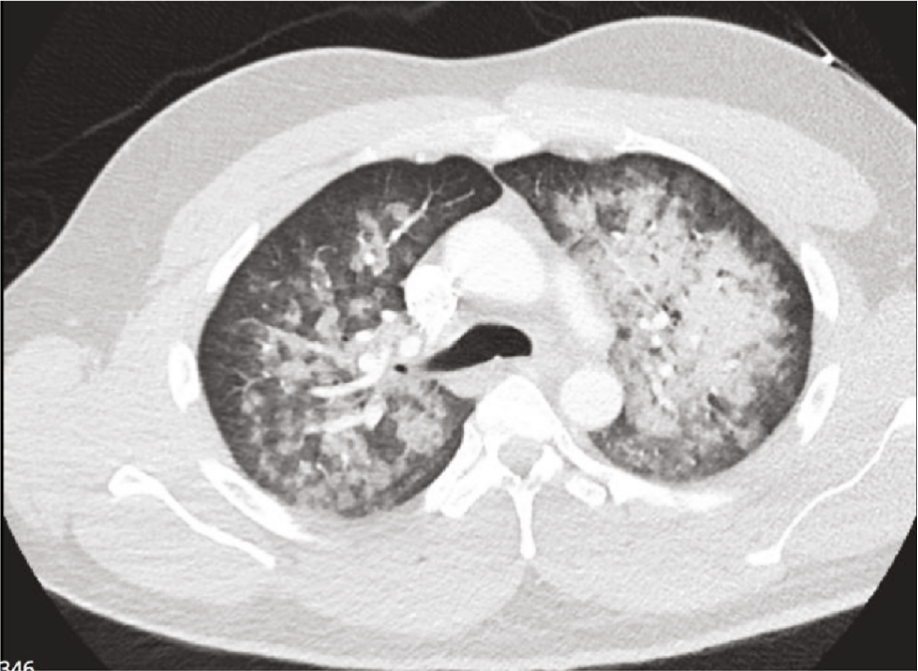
Contrast-enhanced computed tomography of the chest on day #2 shows diffuse bilateral airspace disease characterized by groundglass and consolidative opacities, with relative peripheral sparing and perihilar predominance. No pleural effusions or pneumothorax.
